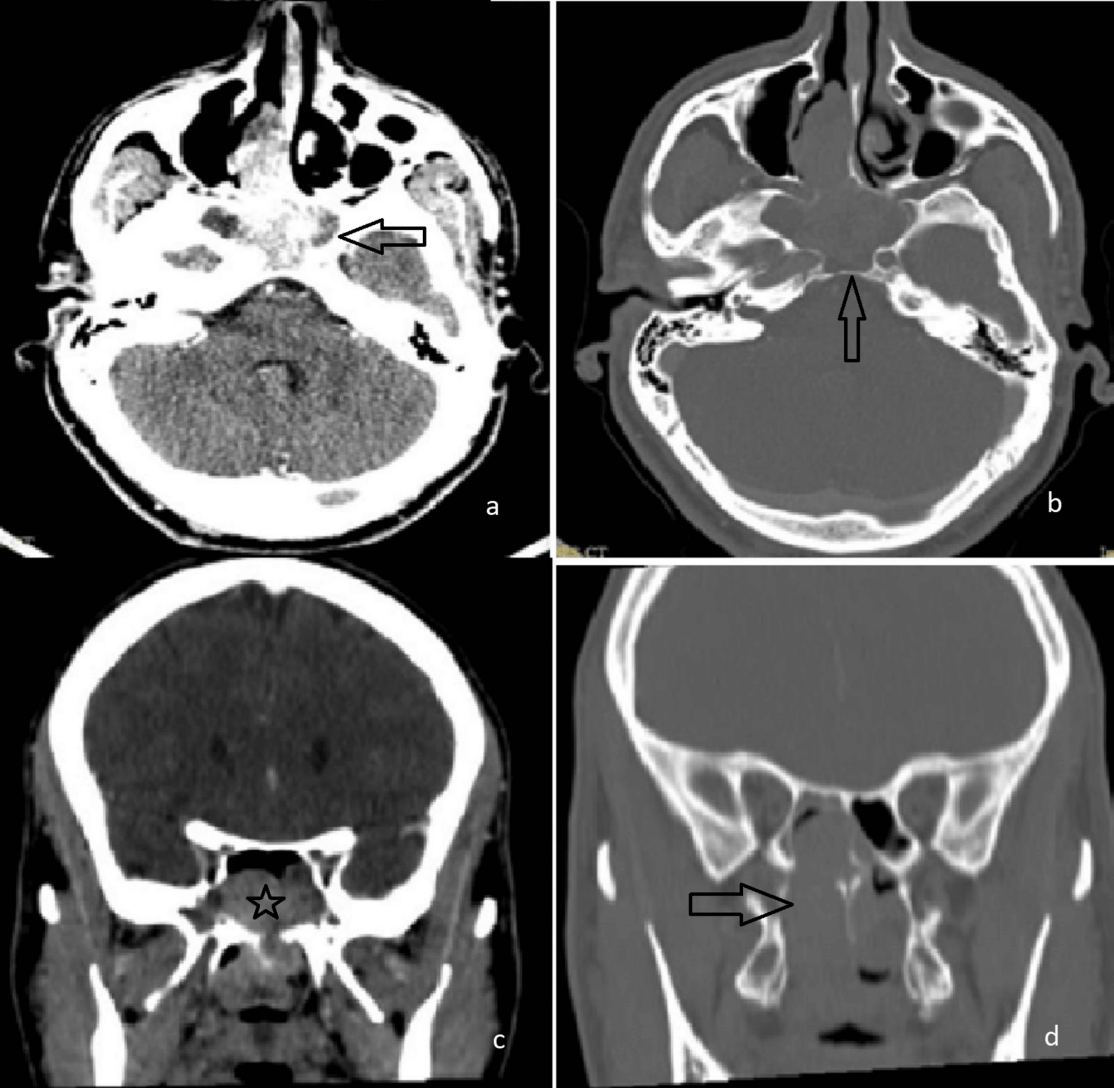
CT scan images of the sphenoid sinus-originated angiofibroma in the patient (a,b) axial views, (c,d) coronal views.
